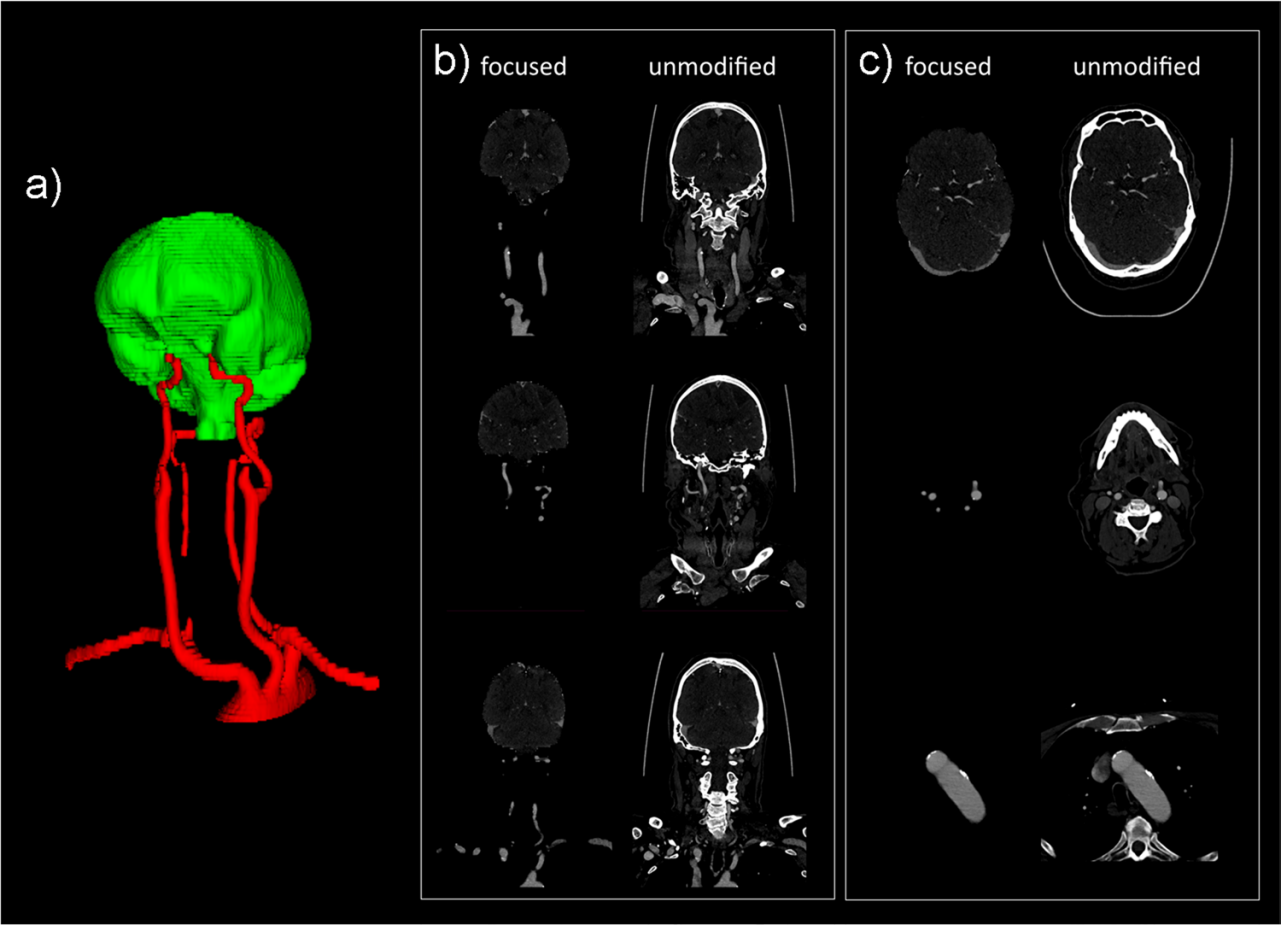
First example of focused view CTA in the test set. Three-dimensional segmentation (a), coronal focused view CTA slices next to unmodified CTA slices (b), and axial focused view CTA slices next to unmodified CTA slices (c) are shown. The full focused view CTA dataset is shown in supplementary (video) file 1
